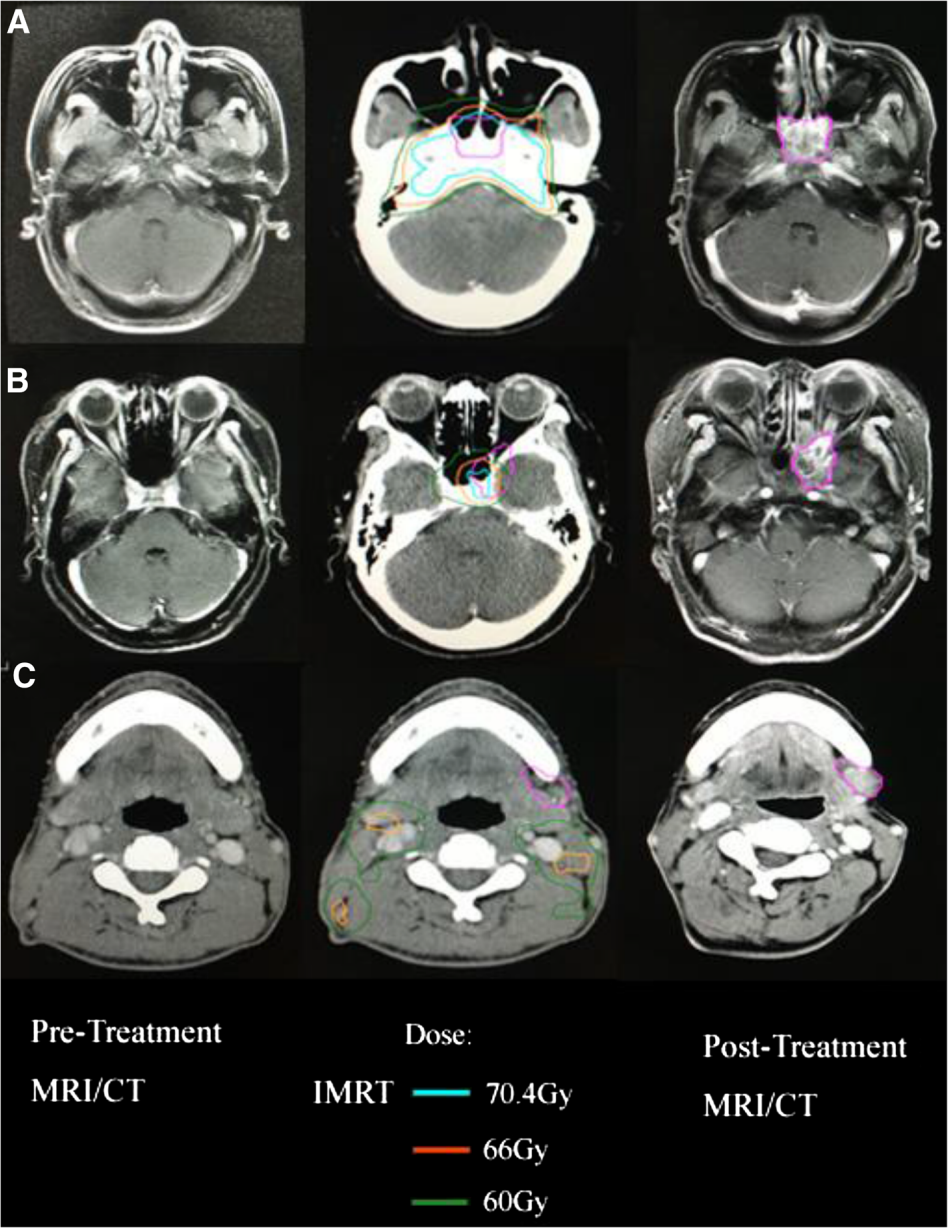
Disease extent for patients having local-regional failures. A, In-field failure. B, Marginal failure. C, Outside-field failure. Left, Pretreatment magnetic resonance imaging (MRI/CT). Middle, The recurrent tumor volumes were transferred from the diagnostic MRI/CT at the time of recurrence to the planning computed tomography to show doses delivered to the recurrence sites. Right, MRI/CT at time of failure. IMRT = intensity-modulated radiotherapy.
